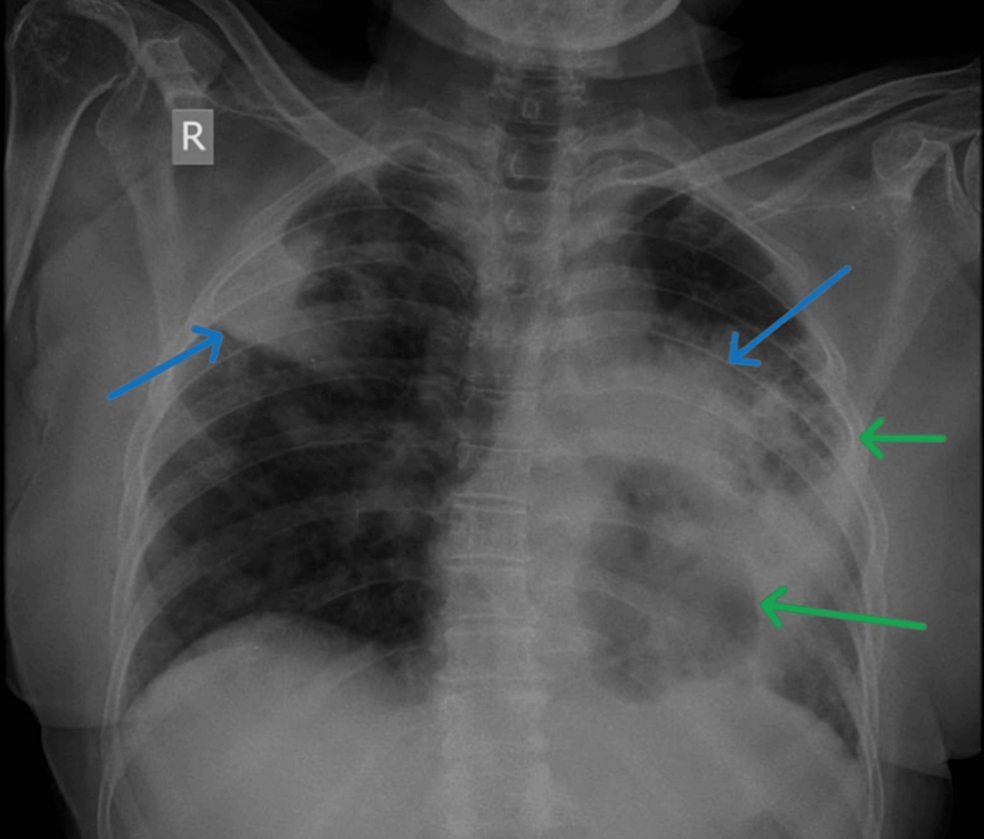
Chest x-ray showing consolidative changes in the right upper zone and left mid zone (blue arrows) with cavitary changes in the left mid and lower zones (green arrows)
